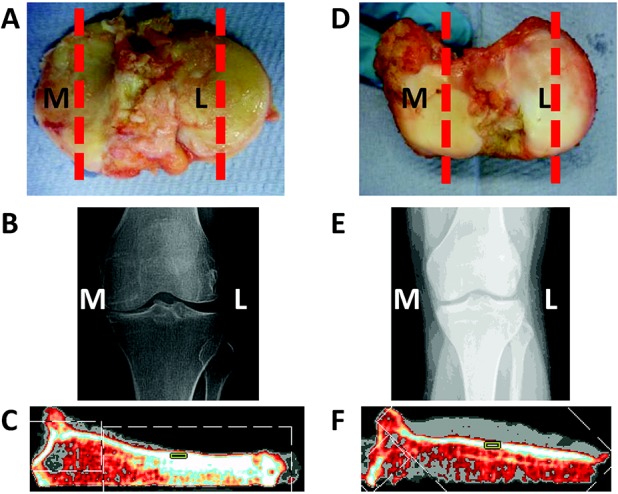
Assessment of the tibial plateau from a patient with osteoarthritis (OA) compared to that from a non-OA control. Postoperative photographs (A and D) and preoperative radiographs (B and E) of the medial (M) and lateral (L) tibial plateaus from a representative patient with OA (A and B) and a non-OA control (D and E) are shown. The medial tibial plateau (C) and lateral tibial plateau (F) from a patient with OA were assessed by peripheral quantitative computed tomography (pQCT). In the pQCT images, the region of interest is demarcated as a green box. The red broken lines in A and D indicate the plane from which the pQCT measurements were obtained.
